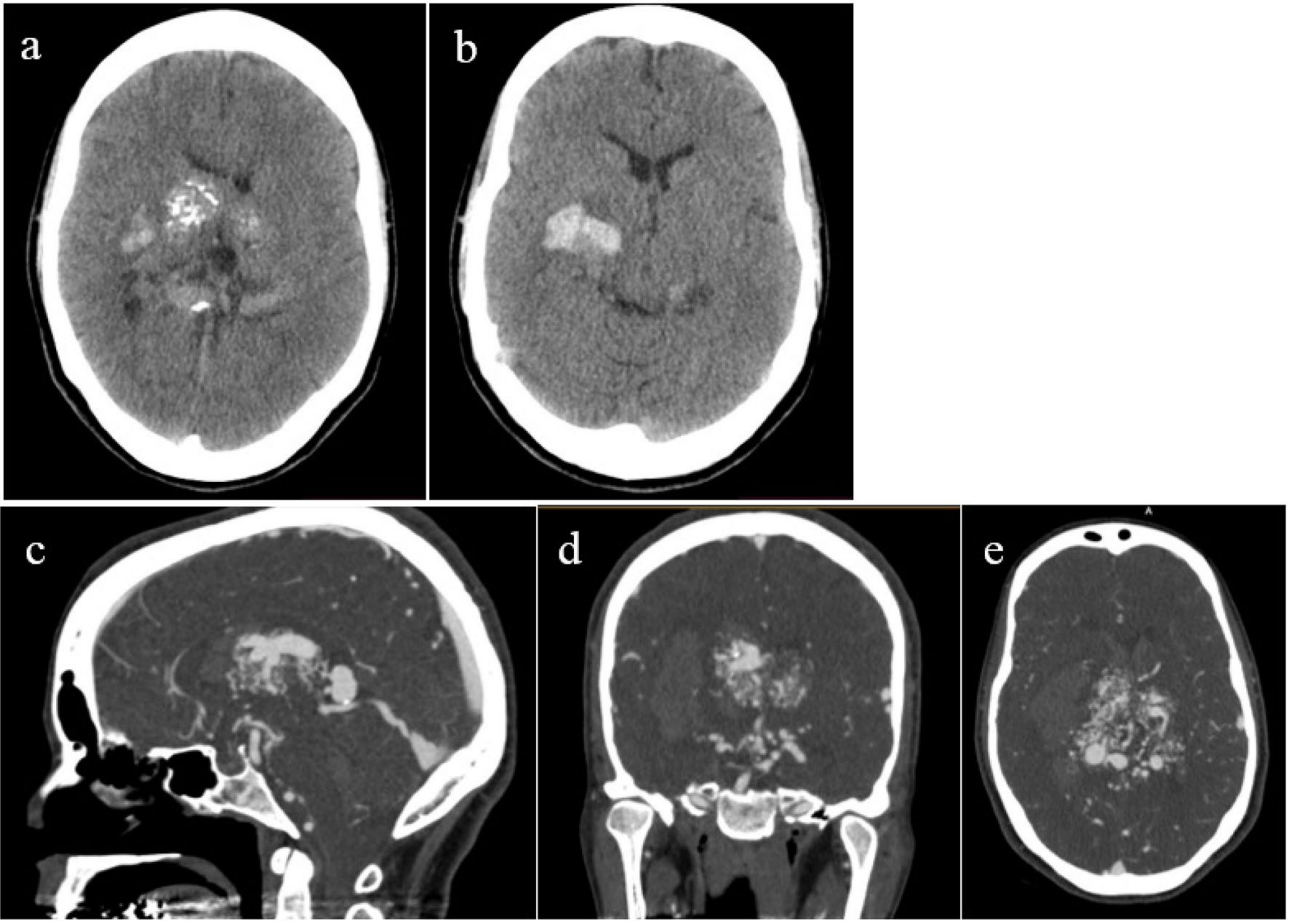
Axial non contrast CT scan show hyperdense lesion with multiple small foci of calcifications in bilateral basal ganglia and hemorrhage in right basal ganglia (a, b). Sagittal (c), coronal (d) and axial (e) cuts of brain CTA show large arteriovenous malformation (AVM) with feeding artery mostly from posterior circulation and draining mostly into the vein of Galen and deep veins.
